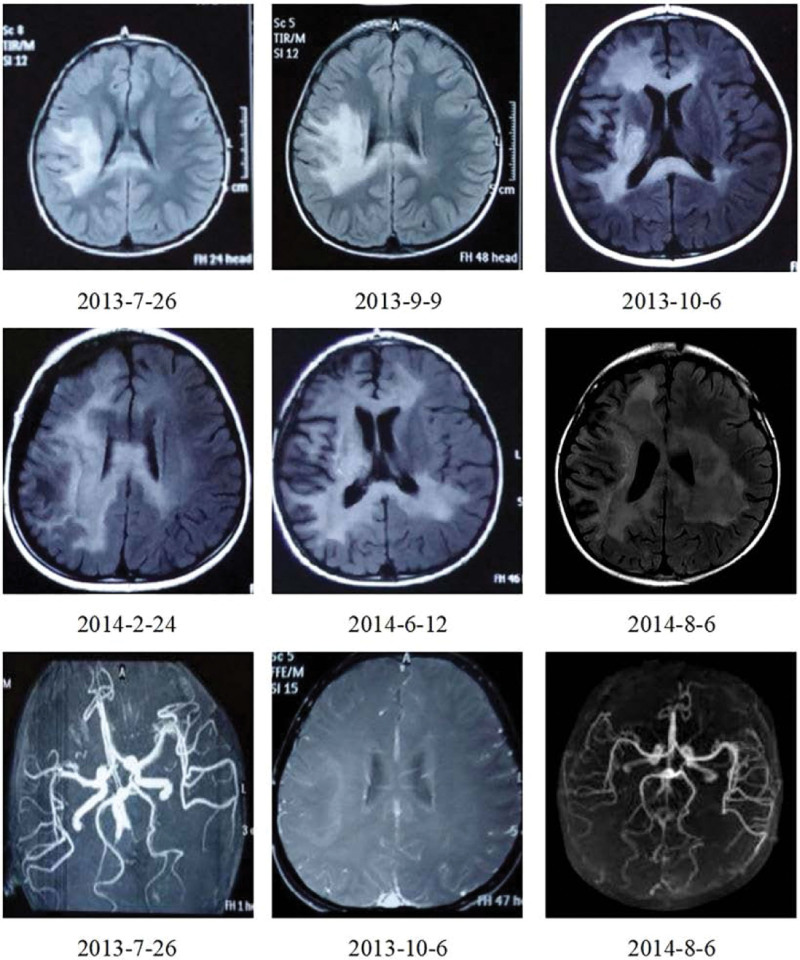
Brain magnetic resonance imaging and angiography: Multiple abnormal signals, enhancement foci in brain parenchyma and meninges, and progression.
